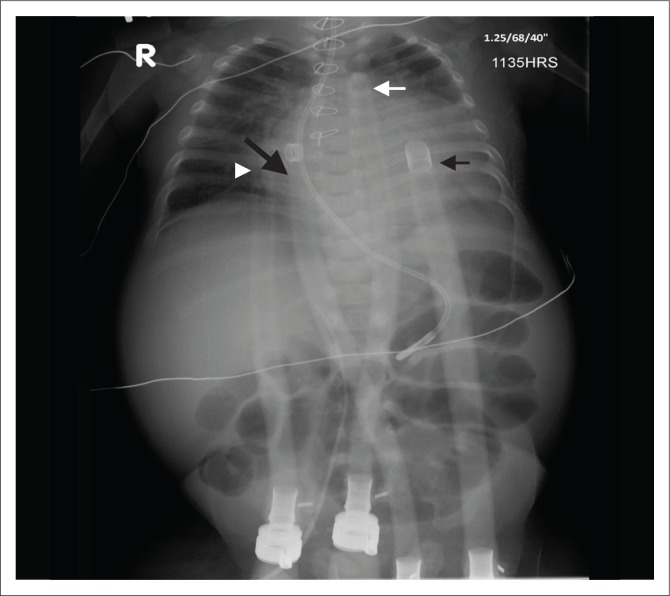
Chest radiograph showing the biventricular assist device (BIVAD) Berlin Heart placed in a 2-week-old infant with severe left ventricular dysfunction and right ventricular hypoplasia. Note the inflow (right black arrow) and outflow cannulas of the left ventricular assist device in the left ventricle and aorta, respectively, while the inflow (arrow head) and outflow (angled arrow) cannulas of the right ventricular assist device are in the right atrium and pulmonary artery, respectively.
